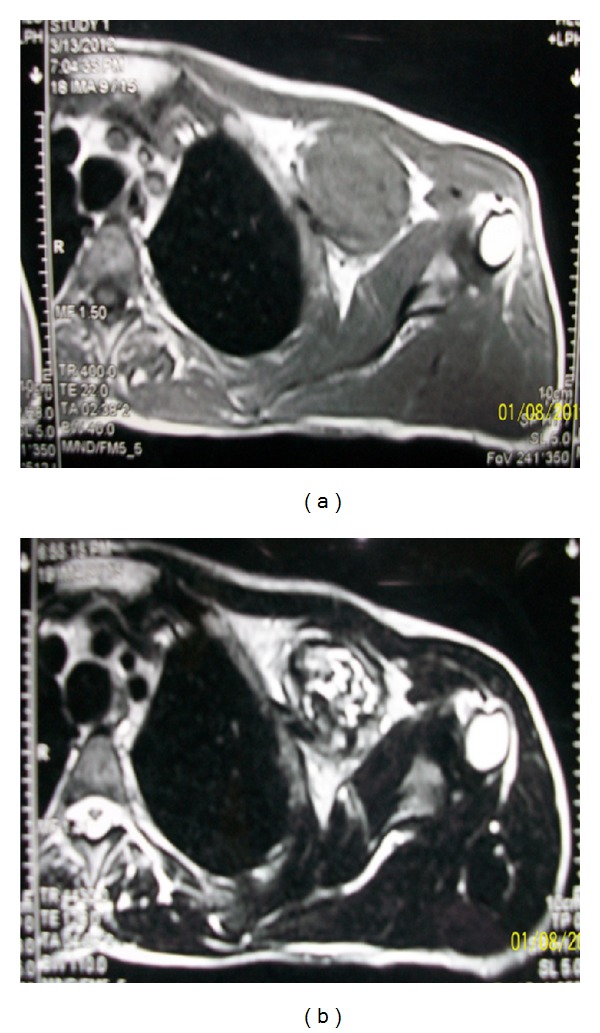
Axial MRI T1W (a) and T2W (b) images showing a well-defined brachial plexus desmoids tumour that was isointense to muscle on T1W sequences and mixed signal intensity (both low to high signal intensity) on T2W sequences and was straddling the brachial plexus.
